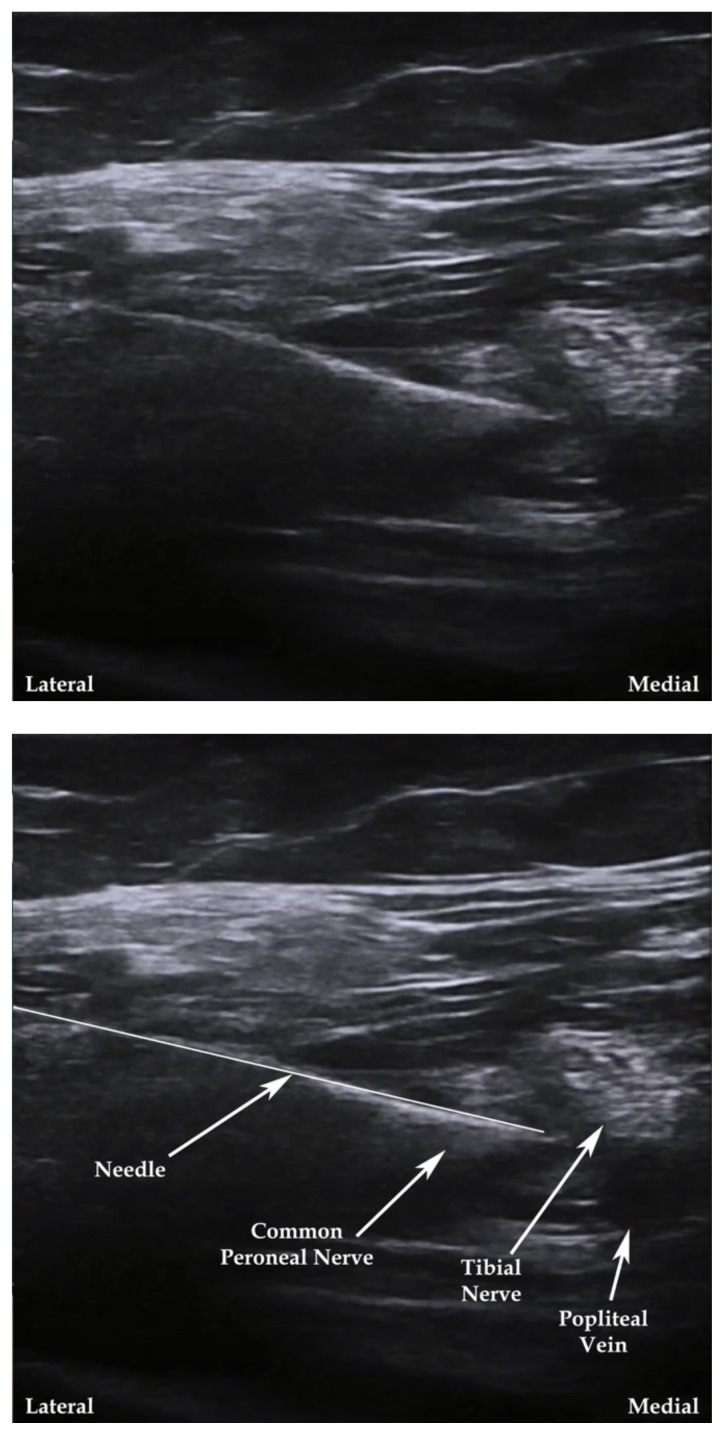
Ultrasound-guided popliteal fossa nerve block. Modified from source.^17^
